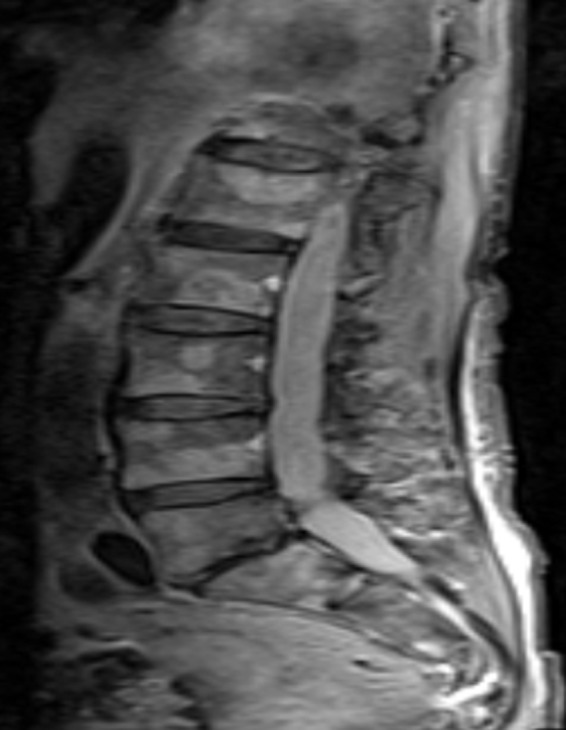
MRI STIR sagittal image of the lumbar spine shows multiple STIR hyperintense lesions. Differentiation between multiple myeloma and metastatic prostate cancer is difficult.
